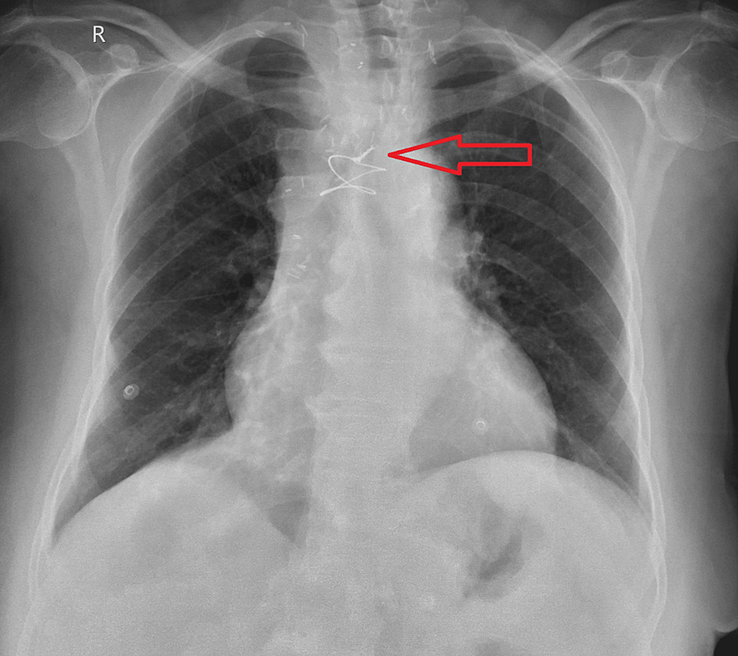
A chest X-ray conducted at the time of admission revealed tracheal deviation to the left side.
